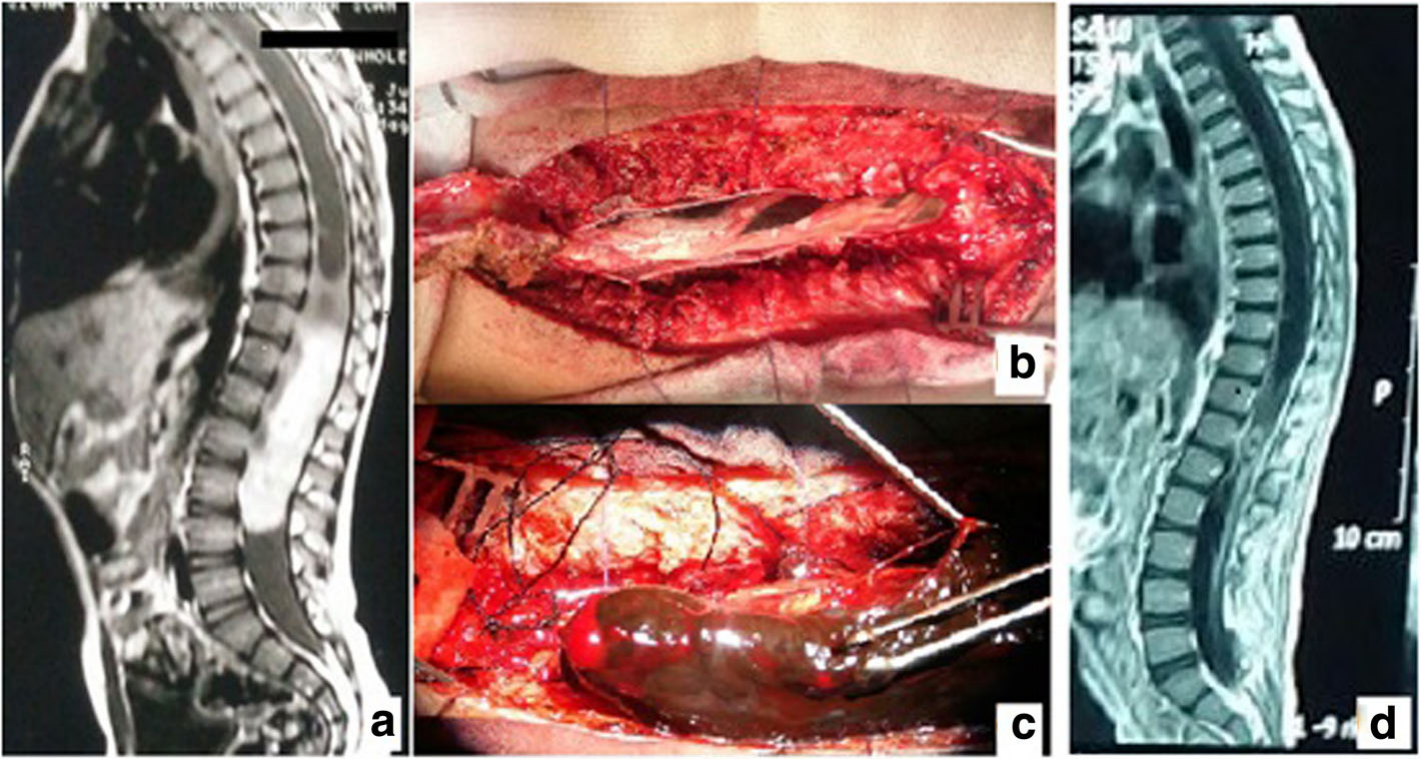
a Preoperative MRI T1WI with contrast. b Intraoperative view with the lesion under the nerve roots. c During tumor removal. d Postoperative MRI T1WI with contrast
